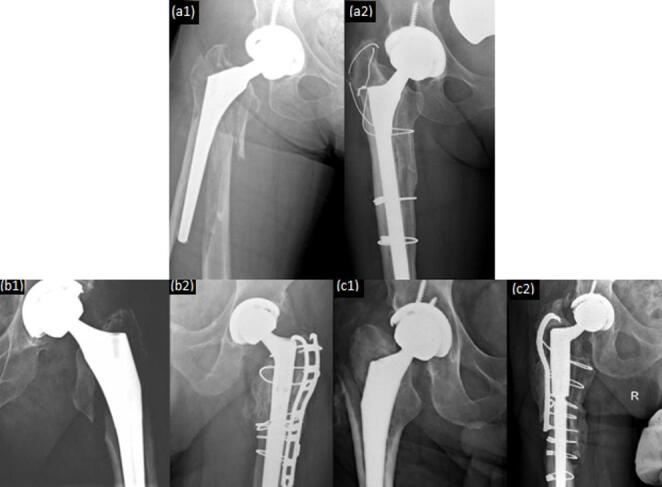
Preoperative and Postoperative Anteroposterior Radiographs. (a1) Fracture fixed first, monoblock (pre-revision). (a2) Fracture fixed first, monoblock (post-revision). (b1) Stem placed first, modular (pre-revision). (b2) Stem placed first, modular (post-revision). (c1) Fracture fixed first, modular (pre-revision). (c2) Fracture fixed first, modular (post-revision)
